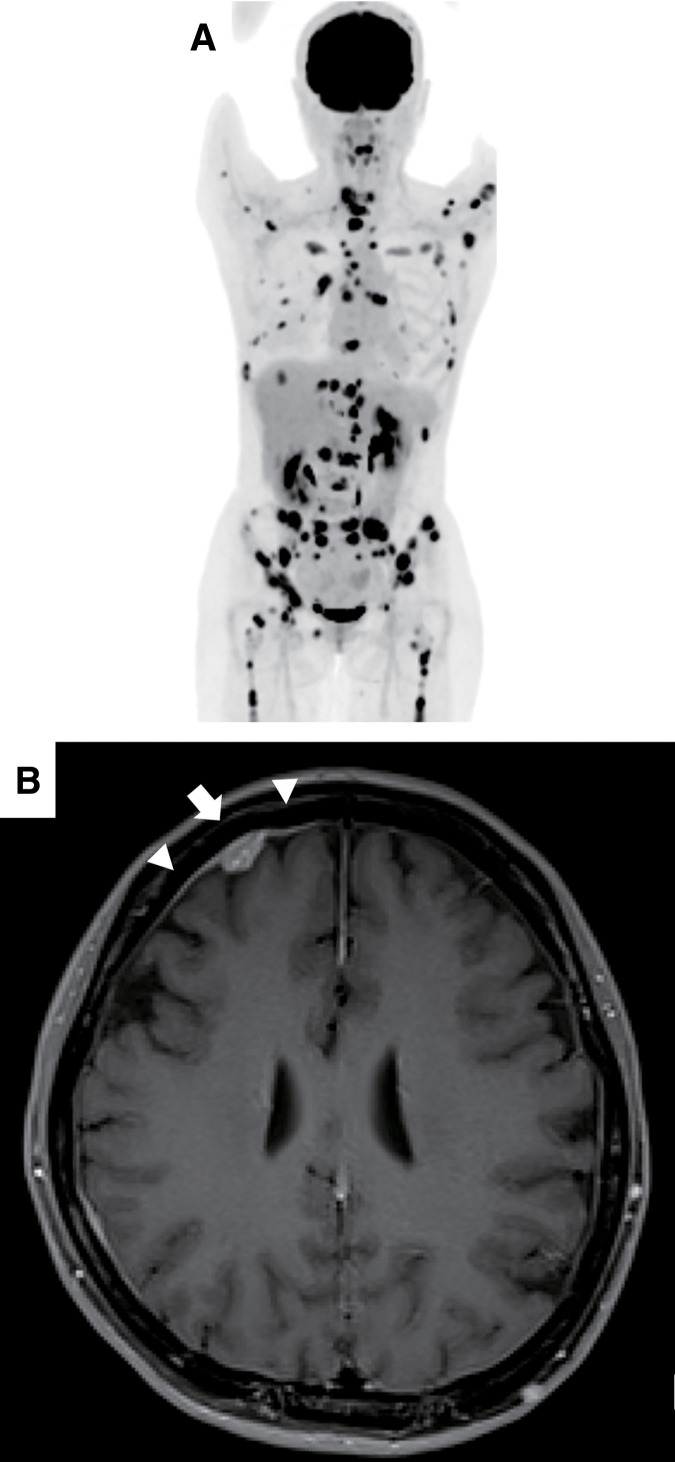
(A) ^18^F-FDG PET/CT showed abnormal uptakes in bone, liver, lung, and distant lymph nodes, indicating multiple systemic recurrences. (B) Gadolinium-enhanced T1-weighted MRI of the brain revealed a nodular enhancing lesion (arrow) and leptomeningeal involvement (arrowheads) in the right frontal lobe, consistent with carcinomatous meningitis.
